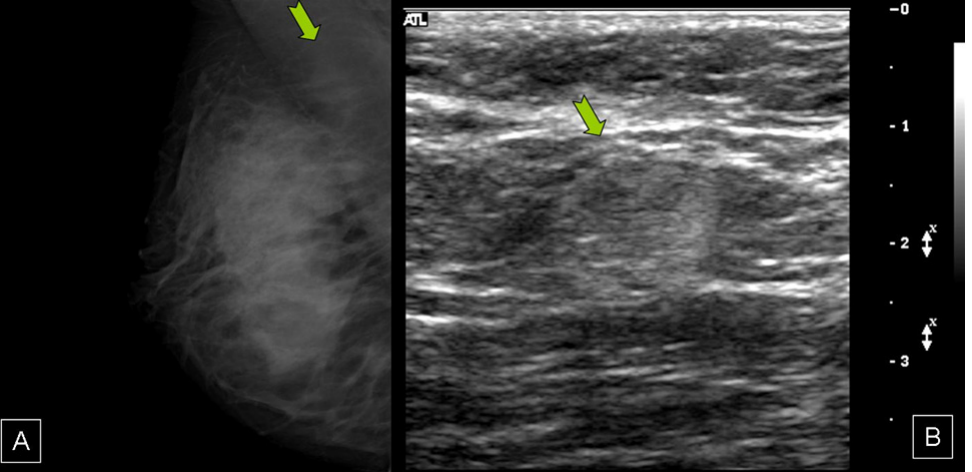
a) Right axillary lymphadenopathy demonstrated on mammogram. b) Ultrasonogram image. The node measures 1 cm and is not clinically palpable.
